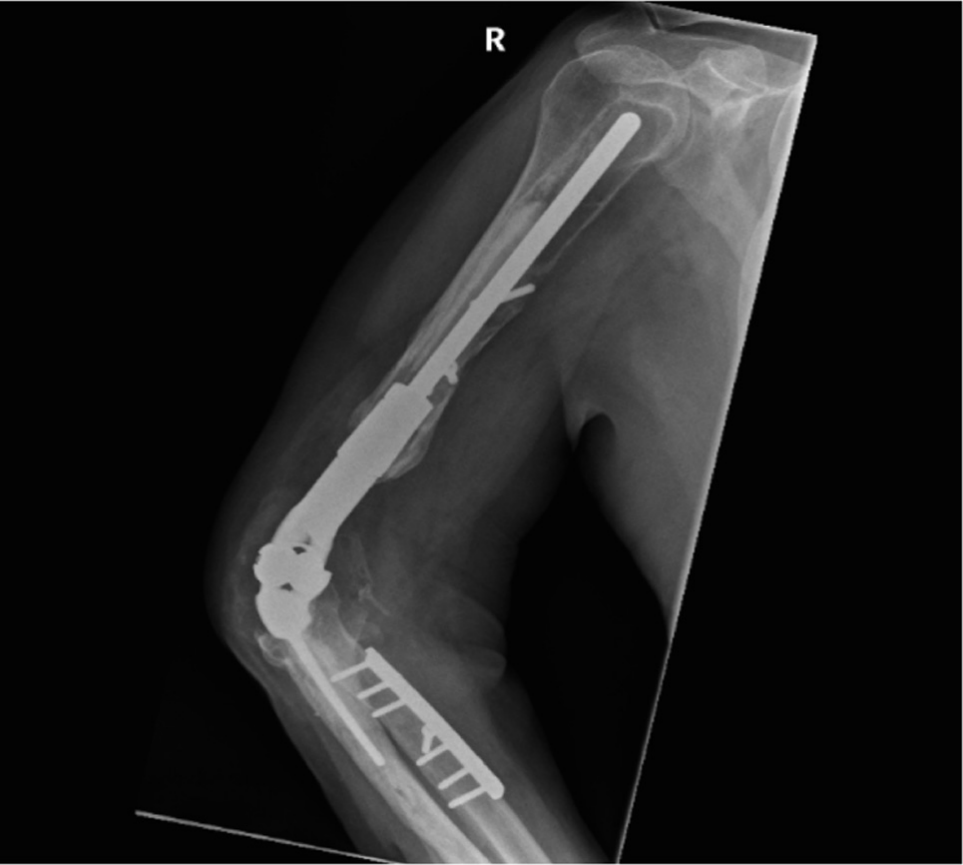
This figure shows the pre-operative radiograph for the first case, demonstrating a complex situation with significant loosening around the stem of a previous endo-prosthetic replacement, with a poor bony envelope remaining.
